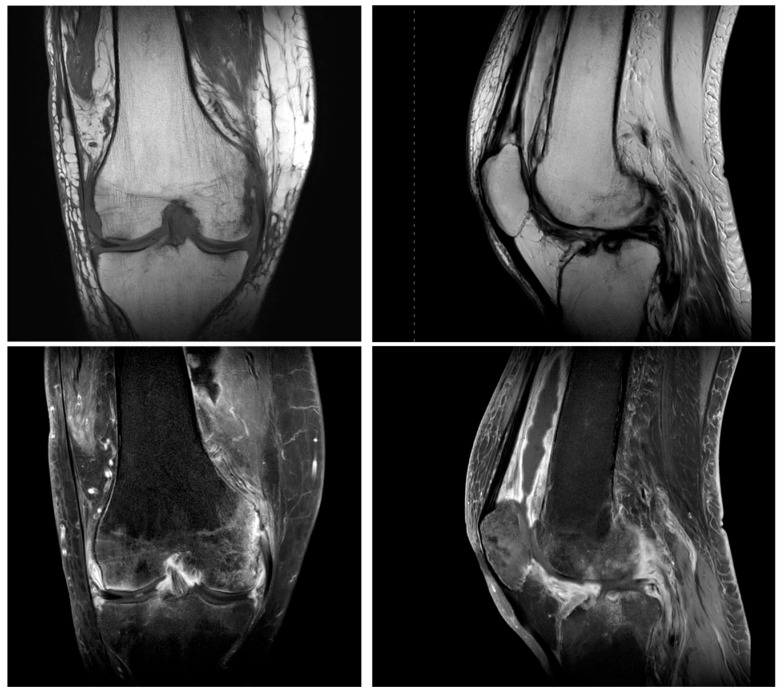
Magnetic resonance imaging showed inflammatory changes in the setting of underlying osteoarthritis.
